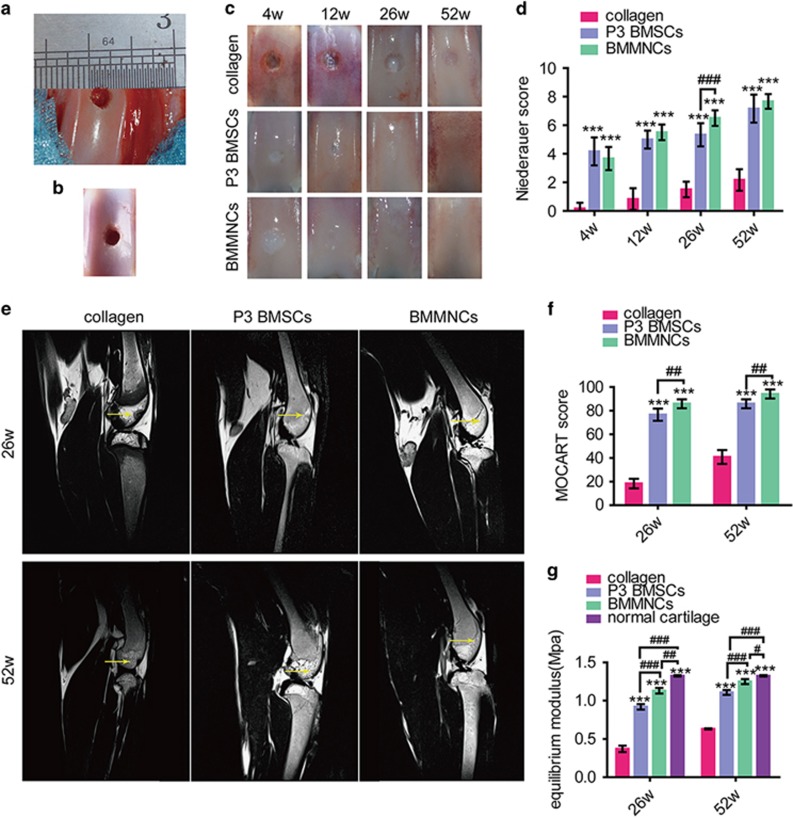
Macroscopic findings, MRI, and mechanical evaluation of repaired cartilage in *vivo*. (a) A 4-mm chondral defect was created in the medial area of the patella groove. (b) Macroscopic finding of the untreated defect at 52 week post-operation. (c) Defects filled with collagen I, BMMNCs/collagen I and P3 BMSCs/collagen I at different post-transplantation time, respectively. (d) Niederauer scoring system were used at each time point; *n*=6. (e) The surface and structure of the repaired tissues were examined by MRI based on the signal intensity of the repaired tissue and the subchondral bone status. (f) MOCART score response to the MRI results; *n*=6. (g) Mechanical evaluation was performed at 26 weeks and 52 weeks after transplantation; *n*=6. Error bars, mean±S.D. *, ^#^*P*<0.05; ^, ##^*P*<0.01; *^, ###^*P*<0.001
